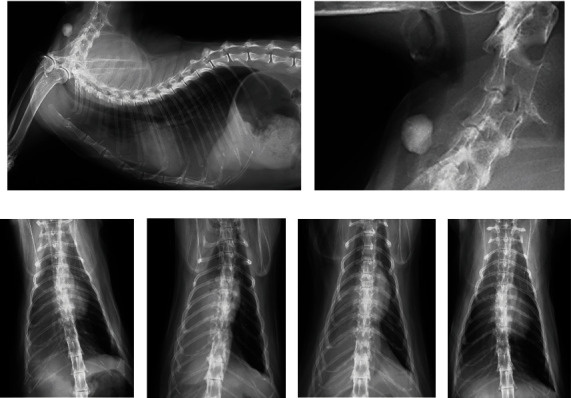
Radiographs of case 2 with a large foreign body (roundish stone) in the proximal trachea. Right lateral cervicothoracic view before surgery reveals increased opacity in the ventral lung field (a) and the stone causing near-complete lumen obstruction (b). Ventrodorsal views demonstrate changes in the right lung opacity before surgery (c), after the first extubation (d), after the positive pressure ventilation before the second extubation (e), and 12 days after surgery (f).
